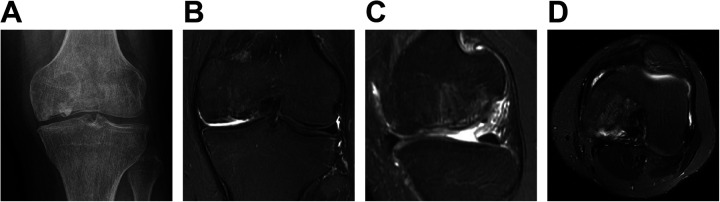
x-ray and MRI after operation at 22 months follow-up. (A) Follow-up x-ray showed no stenosis of the joint space. (B–D) Complete integration of the graft with the bone and articular congruency was observed in MRI.
